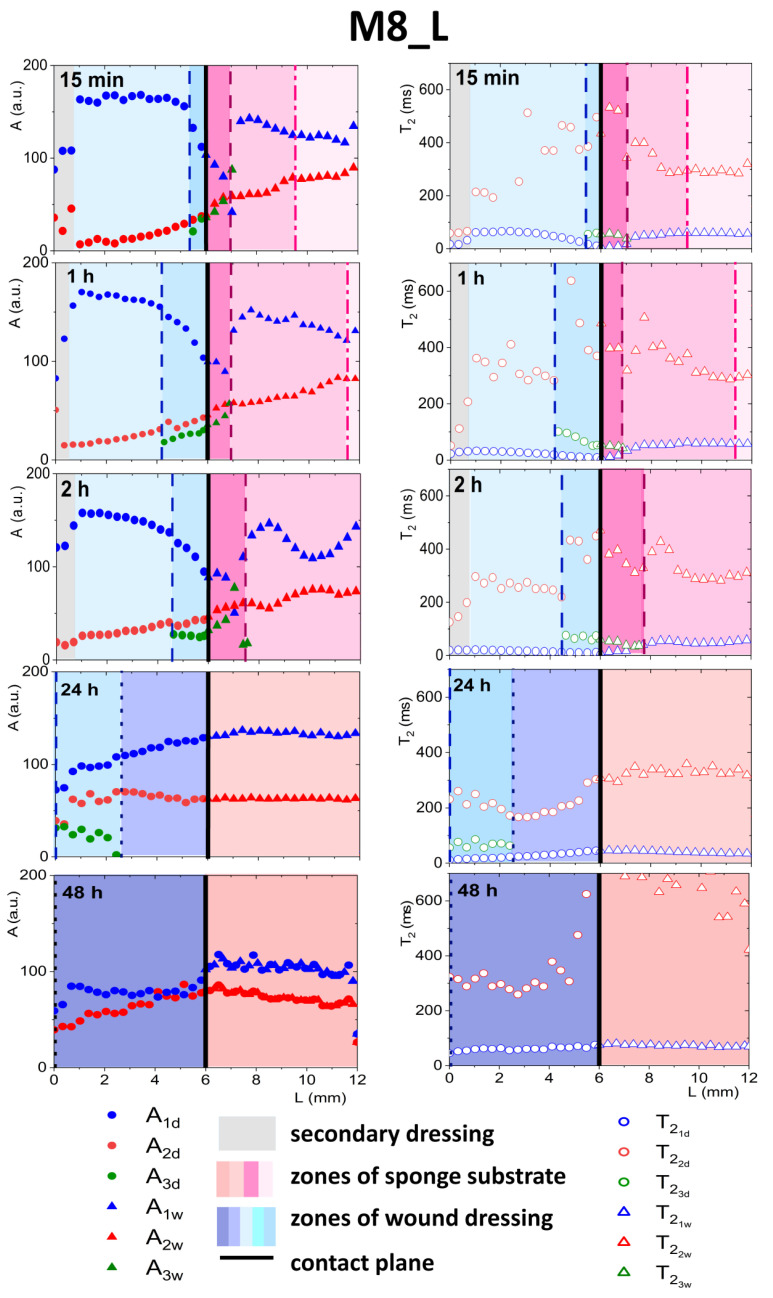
Parameter profiles obtained by MRI, relaxation time (*T*_2_) or signal amplitude (*A*), for M8_L for the whole in vitro wound dressing stack model, when the sponge medium solution was PG.
